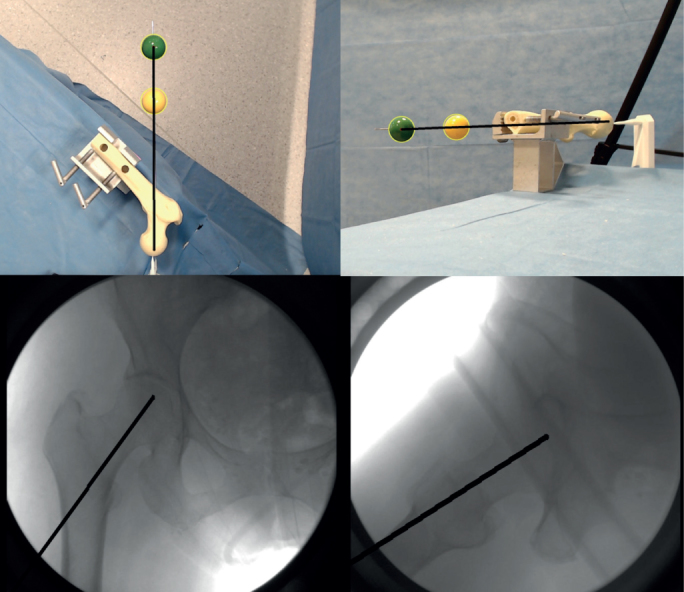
Control screen of the FluoroSim software running with the calibration femur. The software locates the colored markers and finds their center. It marks the position of the guide-wire on the camera image and, using the ATM, overlays this onto the pre-loaded radiograph. Both AP and CTL images are produced.
